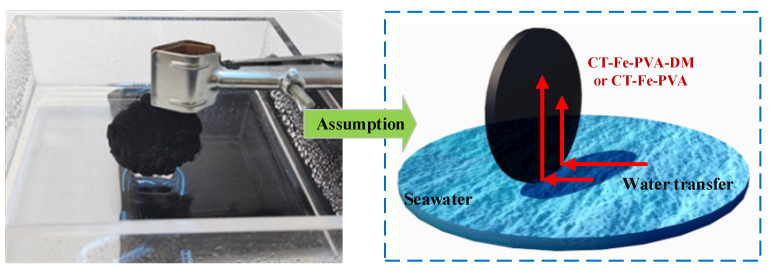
Hydrophilic experimental design of CT-Fe-PVA-DM and CT-Fe-PVA.
